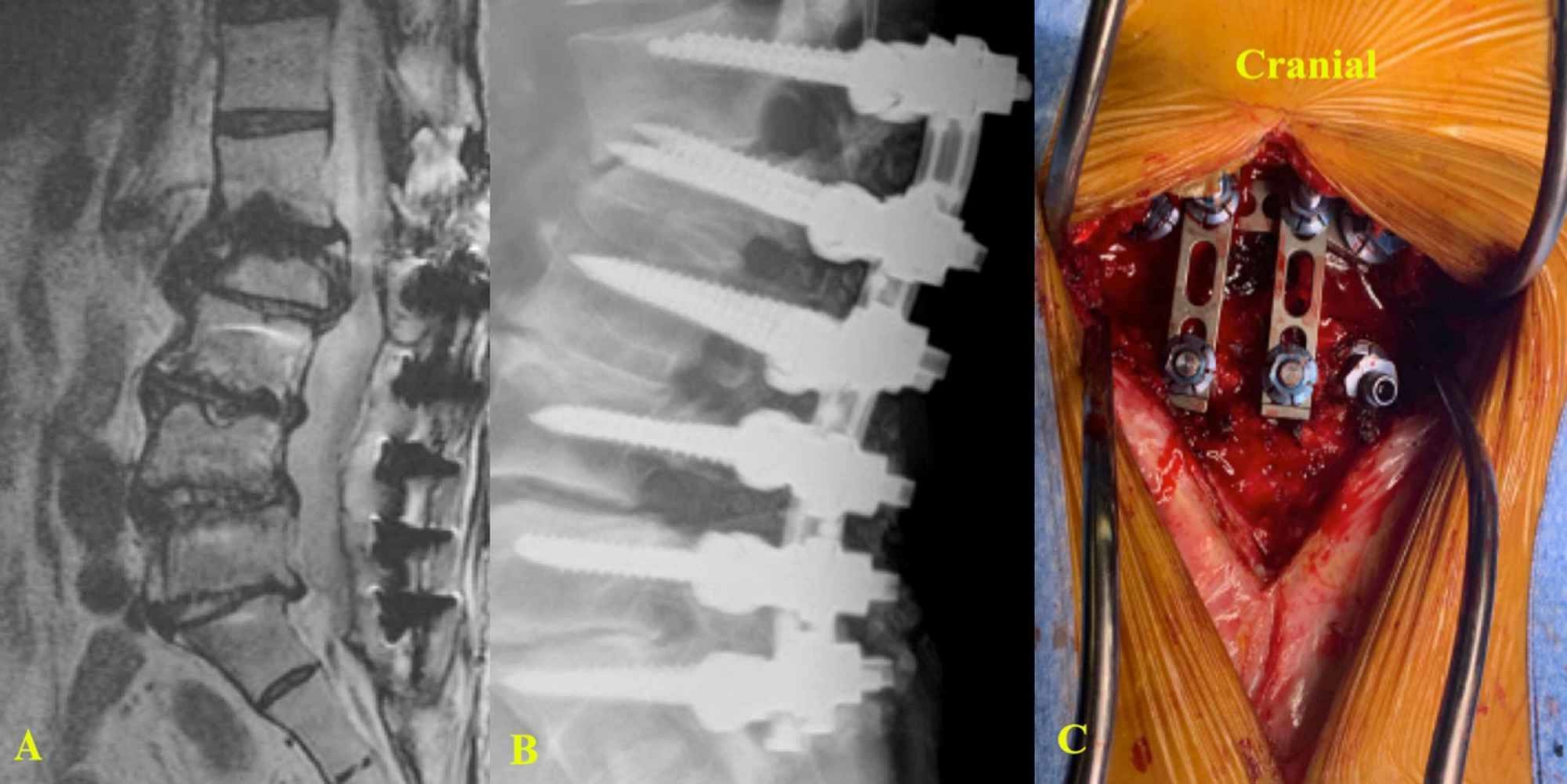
Images of a patient with a previous L2-S1 posterior fusion using the UNIMAX system (A) MRI lumbar spine (sagittal view) shows a new L2 vertebral body fracture. (B) X-ray (lateral view) shows the extension of the construct to L1. (C) Intraoperative picture shows that only partial exposure of the existing construct was necessary to extend the fusion to L1.
